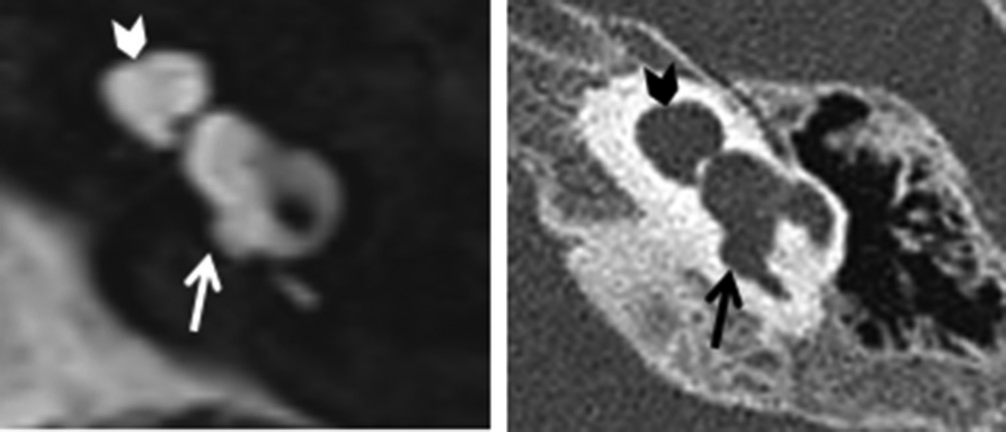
Type 1 incomplete partition appearances on MRI (left) and CT (right), the cochlea has normal dimension and no internal partitioning (arrowheads), the vestibule and semi-circular canals are dysmorphic (arrows).
